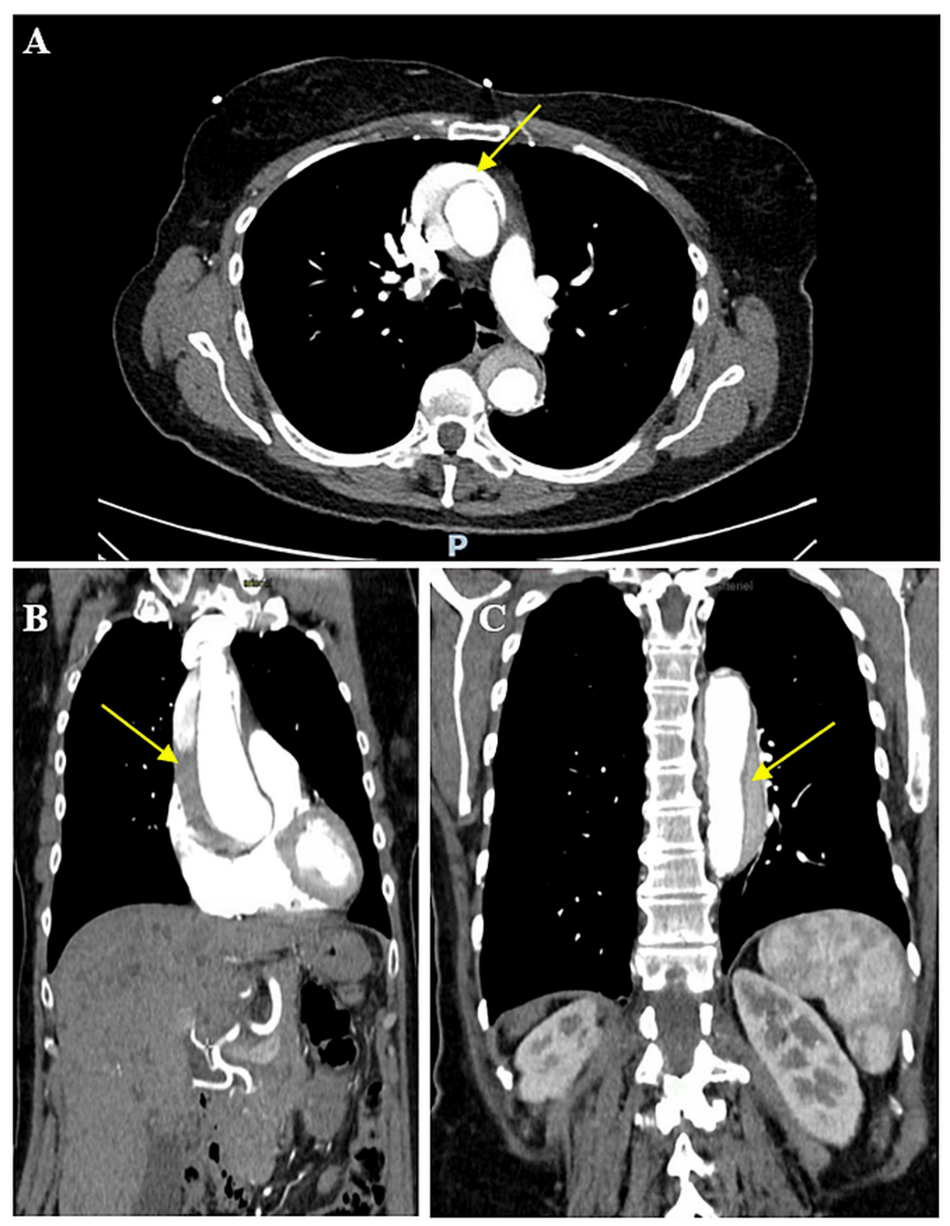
CT angiogram with the axial (A) and coronal (B, C) views showing a Stanford type A thoracoabdominal aortic dissection originating from the focal ulceration of the aortic root, with a spontaneously mural hyperdense hematoma extending 6 cm in an anteroposterior direction. The intimal flap (yellow arrow) extends from the thoracoabdominal aorta to the iliac bifurcation and right external iliac artery CT: computed tomography
